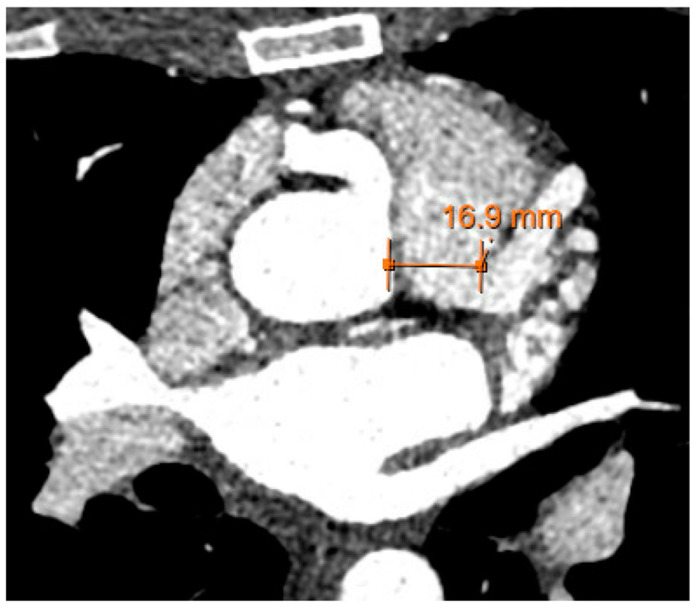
Coronary CT angiogram demonstrating anomalous origin of the left main coronary artery arising from the main pulmonary artery with a dilated right coronary artery.
